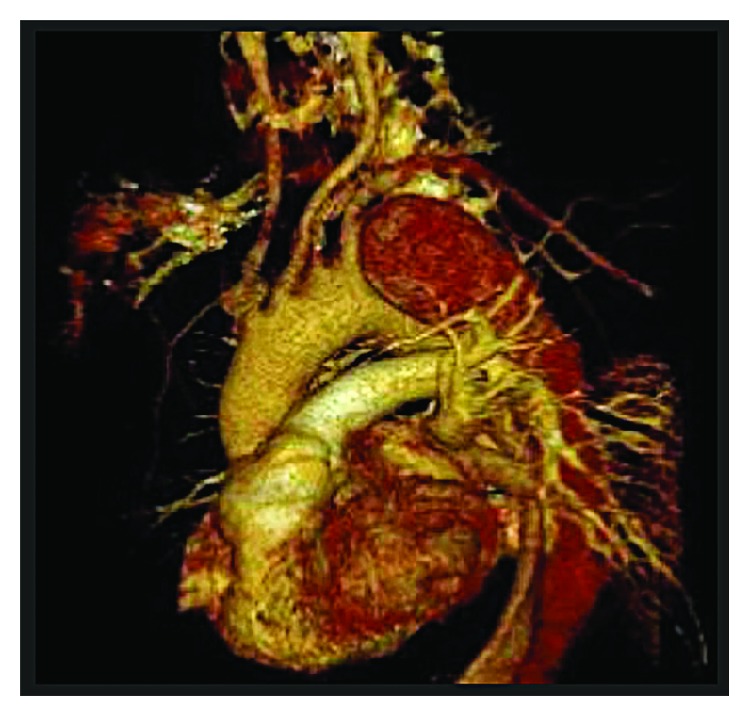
Preoperative virtual 3D CT angiogram of the aorta showing the separate origin of the left vertebral artery from the aortic arch.
